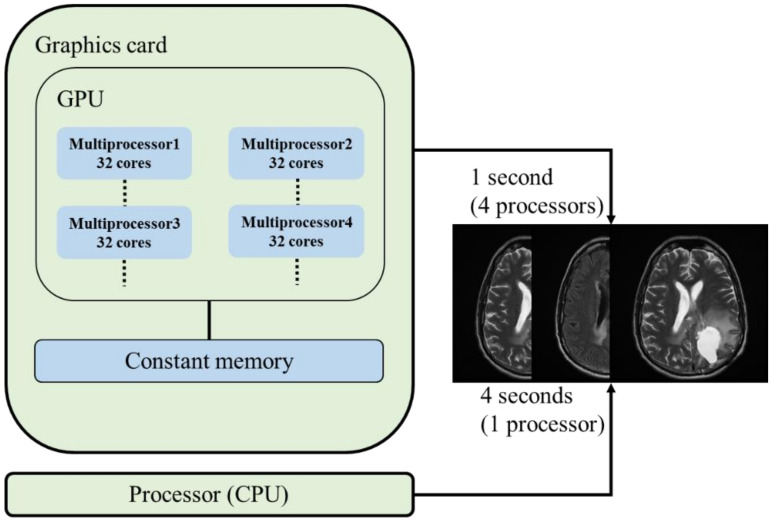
Typical CPU and GPU for brain MRI processing.
